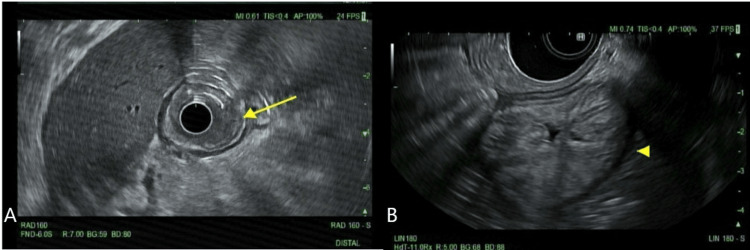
An Ulcerated Mass of the Excluded Gastric Antrum Visualized on Endoscopic Ultrasound (EUS) Shown above are images captured during EUS. The arrows in Figure 3A-3B point to the gastric mass and show endosonographic evidence suggesting invasion into the muscularis propria manifested by abutment
